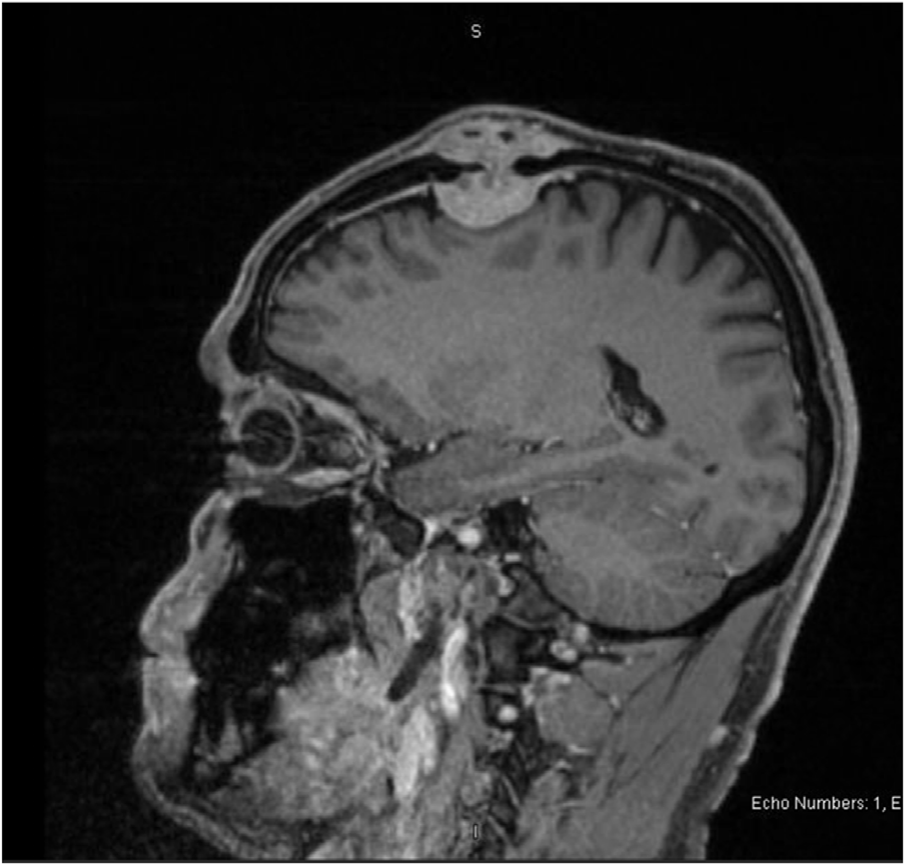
MRI- Sagittal plane. MRI shows a well defined rounded extra-axial lesion seen in the left frontal area measuring 32 × 26 × 18.5 mm in AP transverse and craniocaudal dimension, it is connected to a superficial subcutaneous scalp soft tissue swelling showing the same characteristics and measuring about 37 × 30 mm in transverse and craniocaudal dimension.
